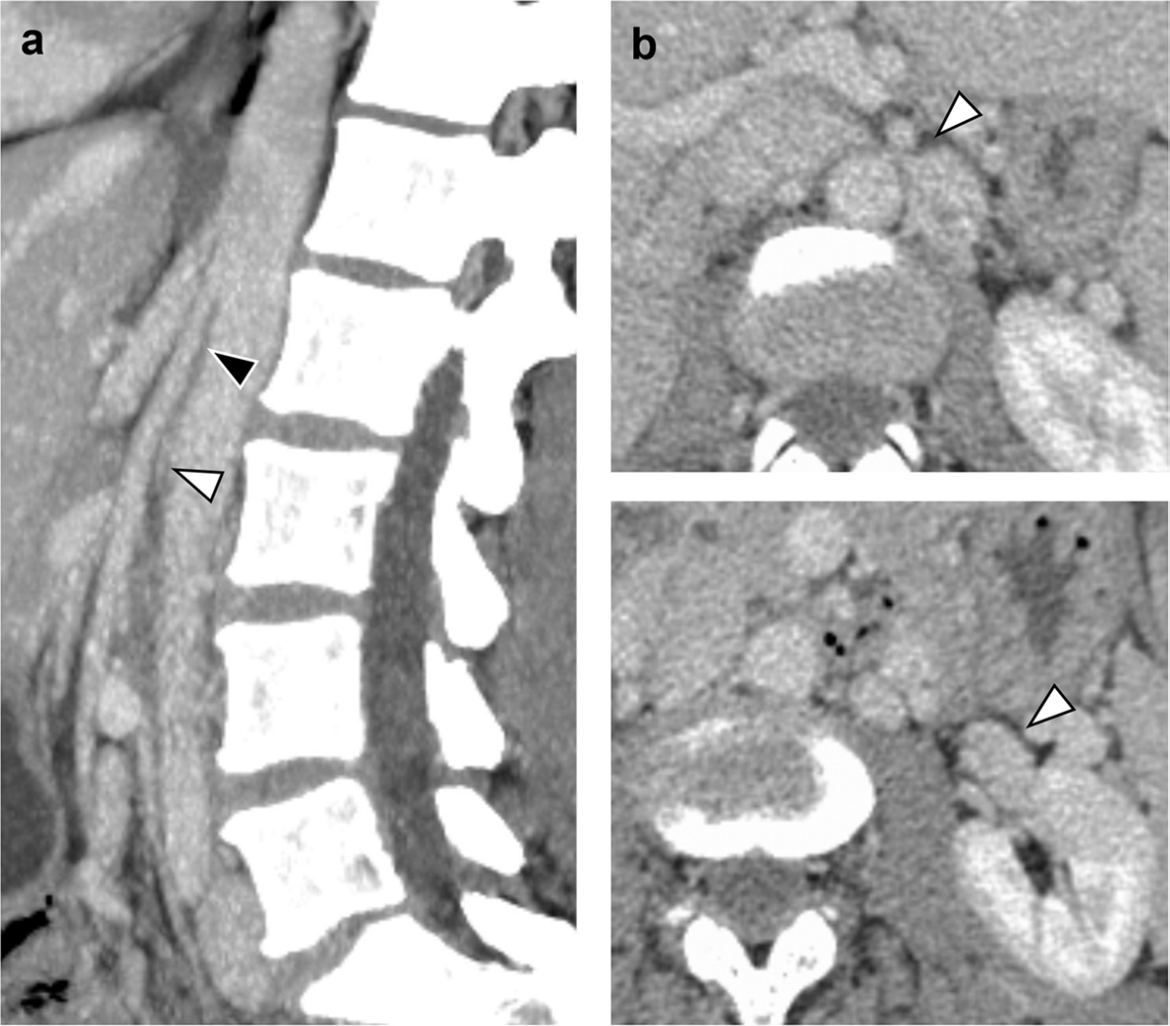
Typical nutcracker anatomy in patient 2. a Sagittal reformatted CT shows a near-parallel course of the proximal SMA (black arrowhead) and the aorta. Note the slit-like compression of the LRV (white arrowhead) b Axial slices depict a focal narrowing of the aortomesenteric portion of the LRV with „beak sign “ and a hilar-to-aortomesenteric diameter ratio of > 5
